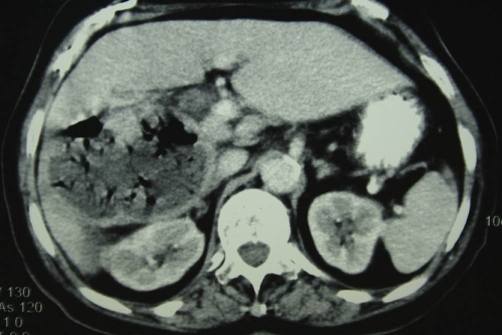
Contrast enhanced CT sections at approximately the level of the right subhepatic space. The right liver lobe cystic lesion contains multiple air-bubbles producing artifacts.
